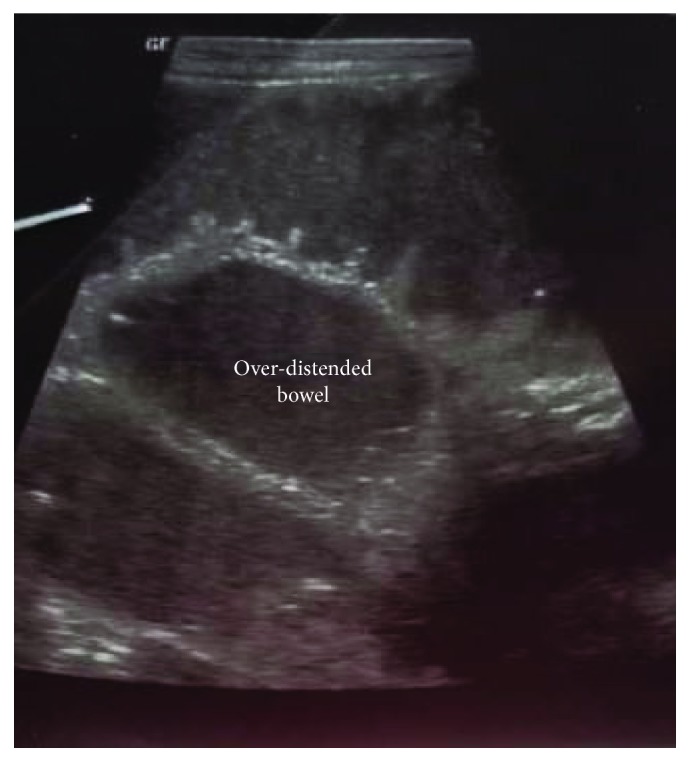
Abdominal ultrasound scan showing the over-distended bowel in the left anterior abdominal wall.
